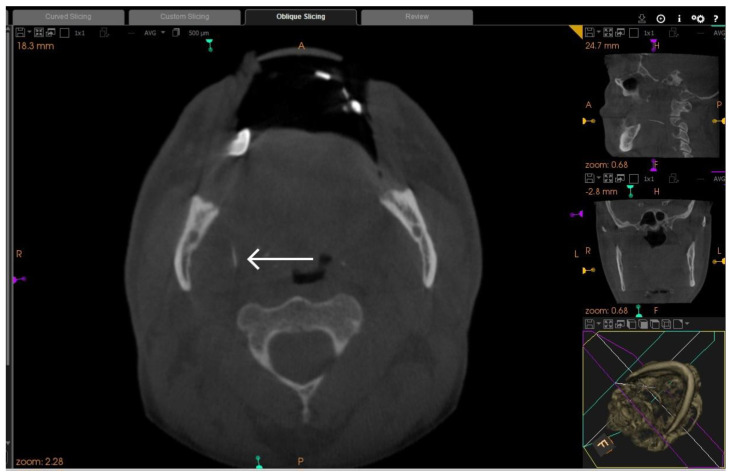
Axial section of CBCT taken five days later showing the anterior part of fractured needle (arrow) in a more posterior position compared to first CBCT image. The needle moved to a deeper location, more inferiorly and medially to the medial pterygoid muscle approaching the junction with lateral pharyngeal space, which necessitated removal under GA. An appointment was given to arrange for removal of the needle under LA and IV sedation after five days. Preoperative CBCT scan and CT-scan with contrast were requested to evaluate the needle and the soft tissue structures around the fractured part (Figure 2 and Figure 3).
